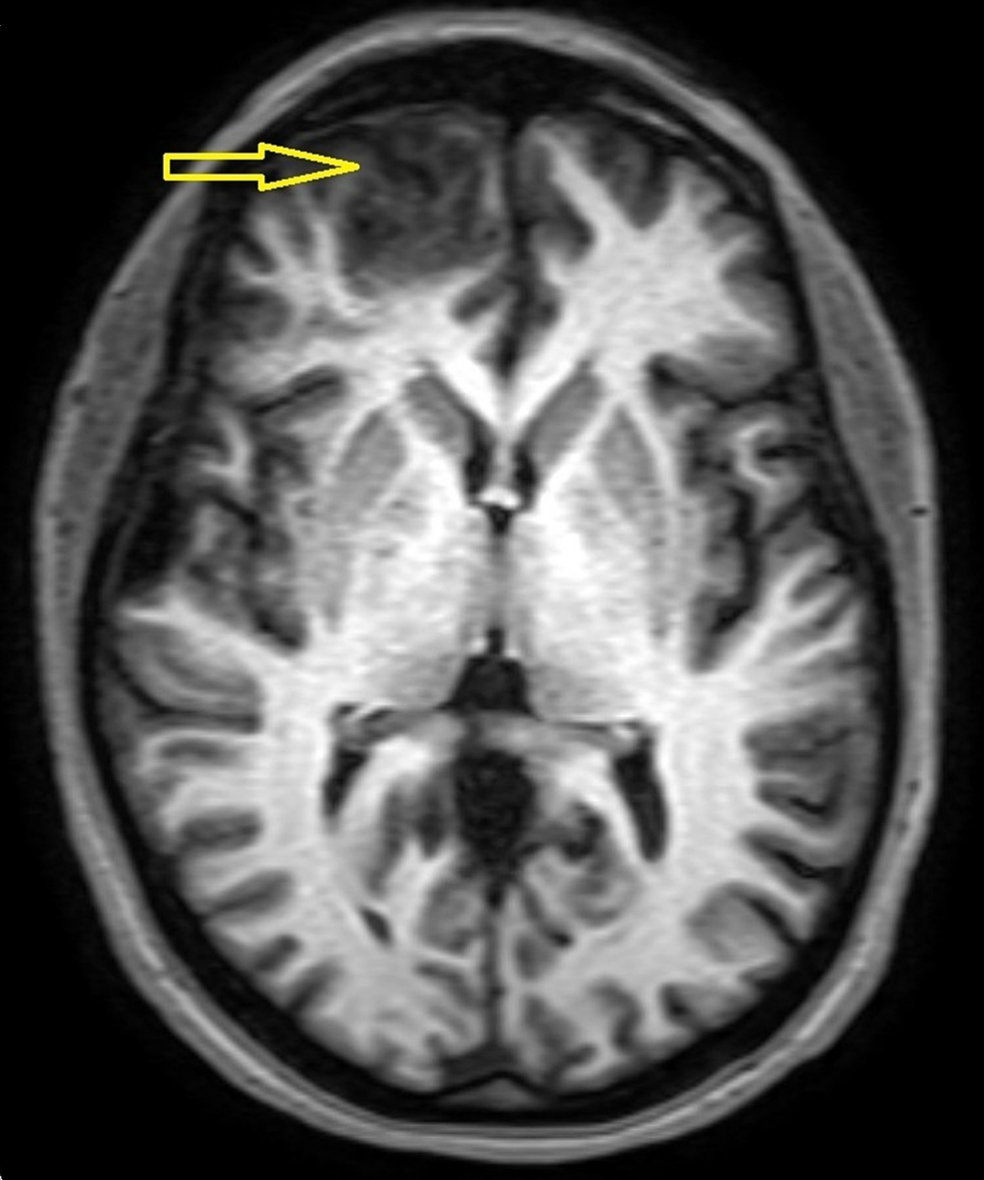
MRI brain indicating the presence of a parenchymal abscess in the right frontal lobe.
